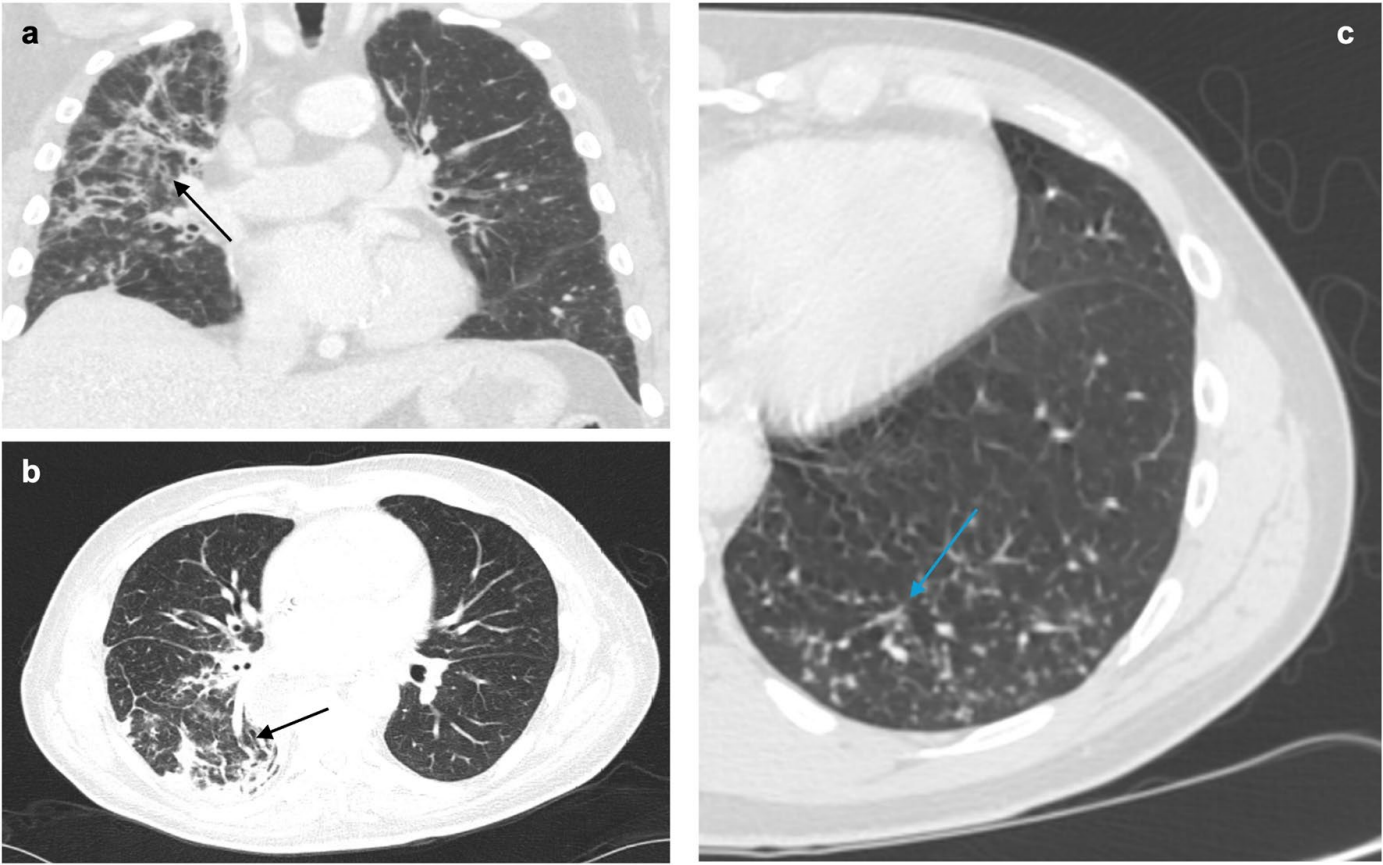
HRCT showing pneumonitis; predominant findings of antineoplastic agent–induced pneumonitis in axial (a) and coronal (b, c) planes are diffuse/multifocal ground-glass opacities (black arrows) or tree-in-bud opacities (blue arrow) with intralobular interstitial thickening and consolidations
